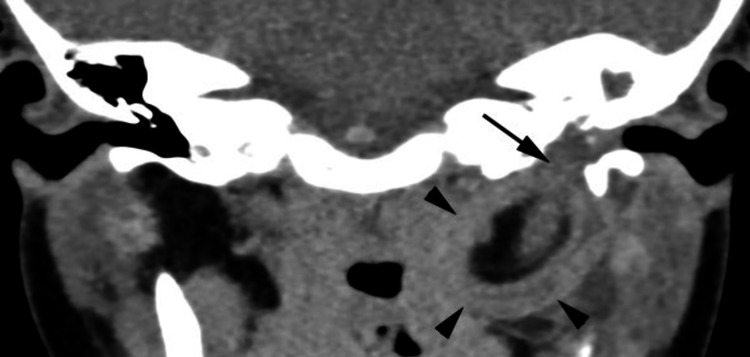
Coronal HRCT temporal (soft tissue algorithm) This CT scan depicts an opacified left middle ear and mastoid antrum with the appearance of an ovoid lesion occupying the left parapharyngeal space (arrowheads) in contrast with the normal right parapharyngeal space. The lesion is heterogeneous with predominantly fatty attenuation centrally, and a laminated soft tissue capsule. Curvilinear soft tissue traversing the fatty central matrix could represent septations. The floor of the left middle ear appears absent (arrow); this is due to the widening of the osseous Eustachian tube seen on axial images (Figure 3). The soft tissue capsule showed minimal enhancement post iodinated contrast administration (not shown) HRCT: high-resolution computed tomography
